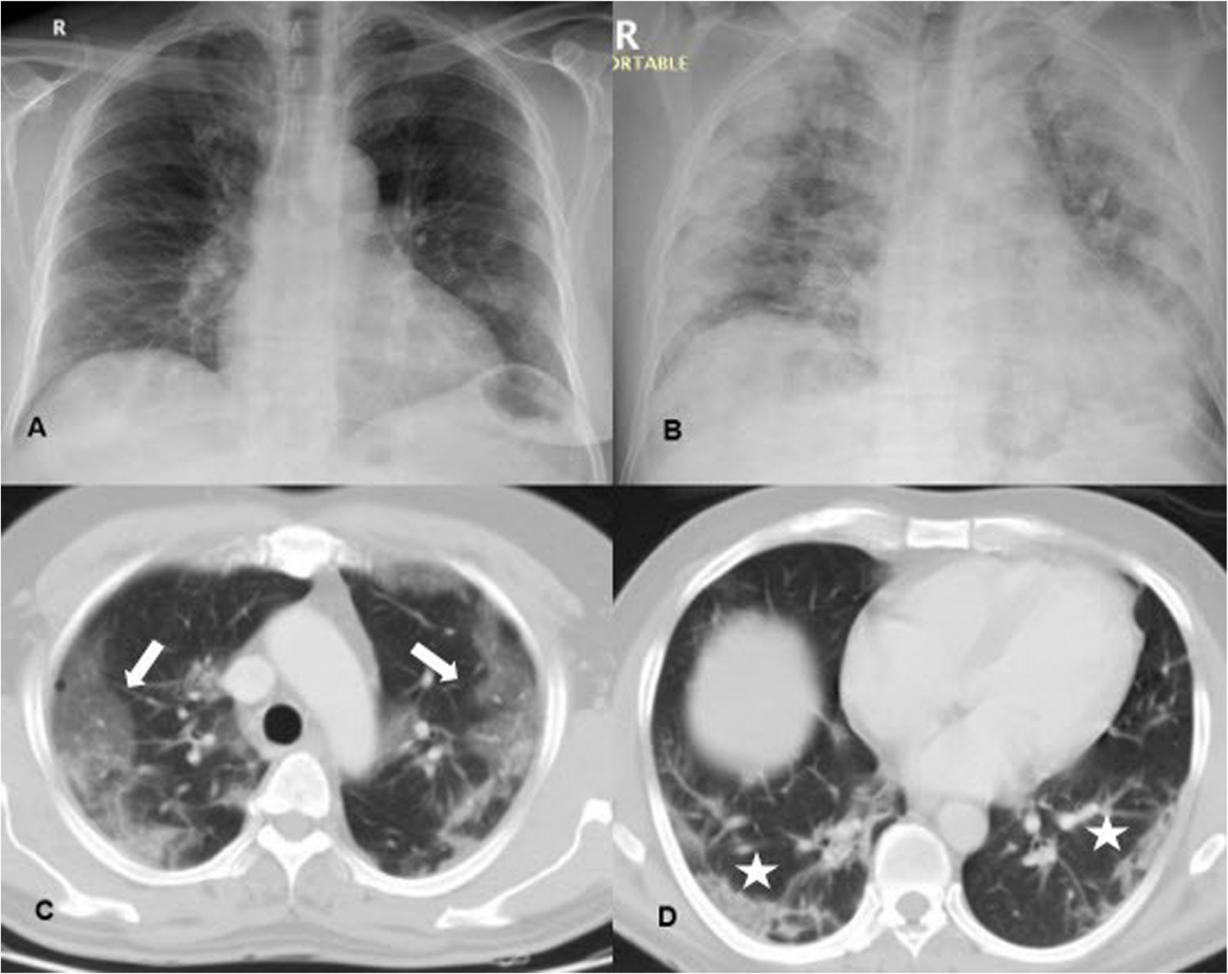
(A) Initial normal chest radiograph of a 70-year-old male who was admitted to our hospital with fever, cough, and dyspnea. (B) Follow-up chest radiograph after 7 days, the patient’s condition deteriorated, and the radiograph revealed extensive bilateral airspace opacities. (C and D) Axial CT images, lung window, on the same day of (B) which revealed multiple bilateral peripheral subpleural GGO (white arrows) and subsegmental consolidations (white stars) indicating a high probability of COVID-19 and severity score 16. The patient was admitted to the ICU and later on RT-PCR was positive
